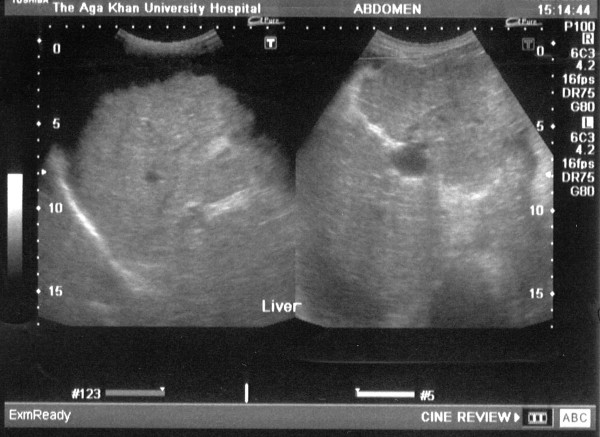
Ultrasound upper abdomen showing coarse liver parenchyma, irregular margins of liver, parahepatic ascities.
